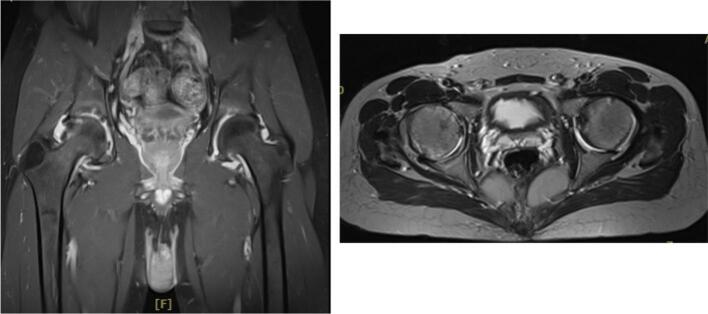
Pre-operative MRI coronal dan axial view (Low signal single band at bilateral femoral head) for patient V.
